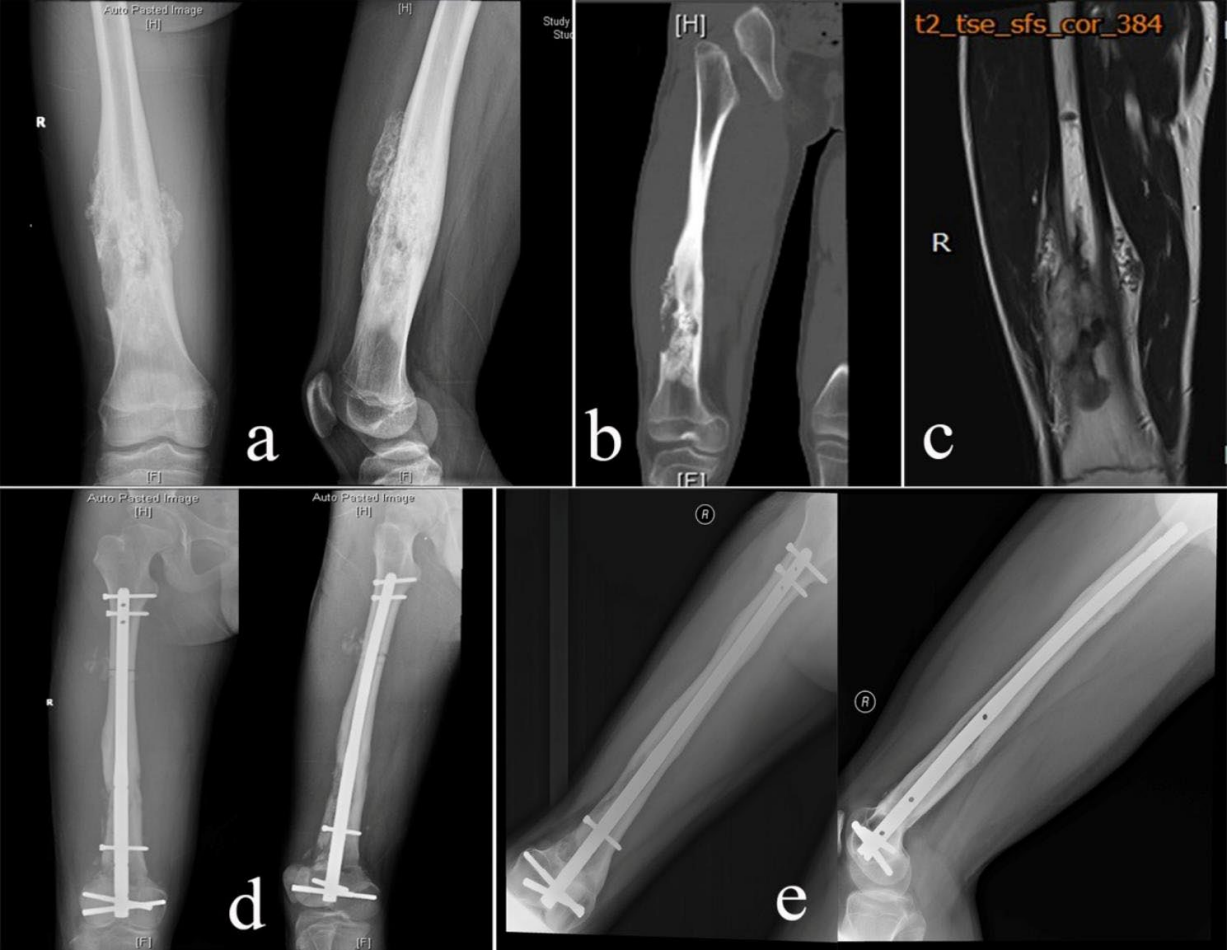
A 16-year-old male with osteosarcoma involving the diaphysis of the right femur (case 24). (a, b and c X-ray, CT and T2-weighted MRI (TR 3000.0ms, TE 98.0ms) showed the tumor involving the diaphysis of the right femur. d Postoperative X-rays after intercalary resection and recycling autograft reconstruction with intramedullary nail fixation. e X-ray at 10 months after reimplantation showing union at both osteotomy sites.)
